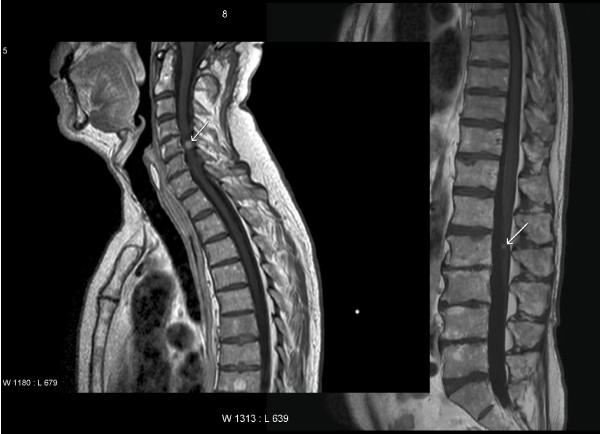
Magnetic resonance imaging (T1-weighted post Gadolinium) showing two of several contrast-enhancing leptomeningeal metastases, indicated by white arrows.
